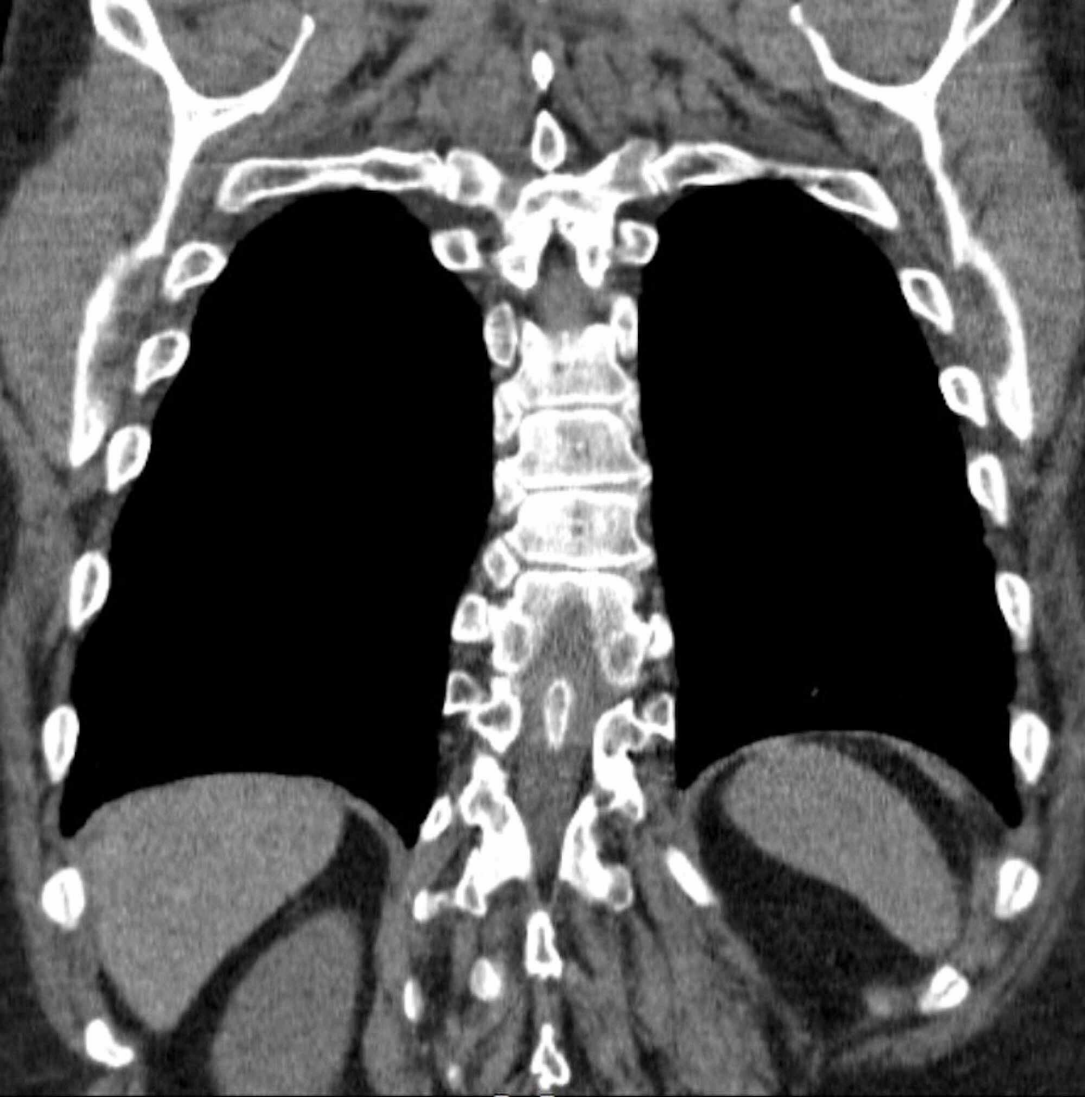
CT of the chest without contrast, coronal view. This shows an osseous structure dividing the vertebral canal in two as well as mild thoracic levoscoliosis.
